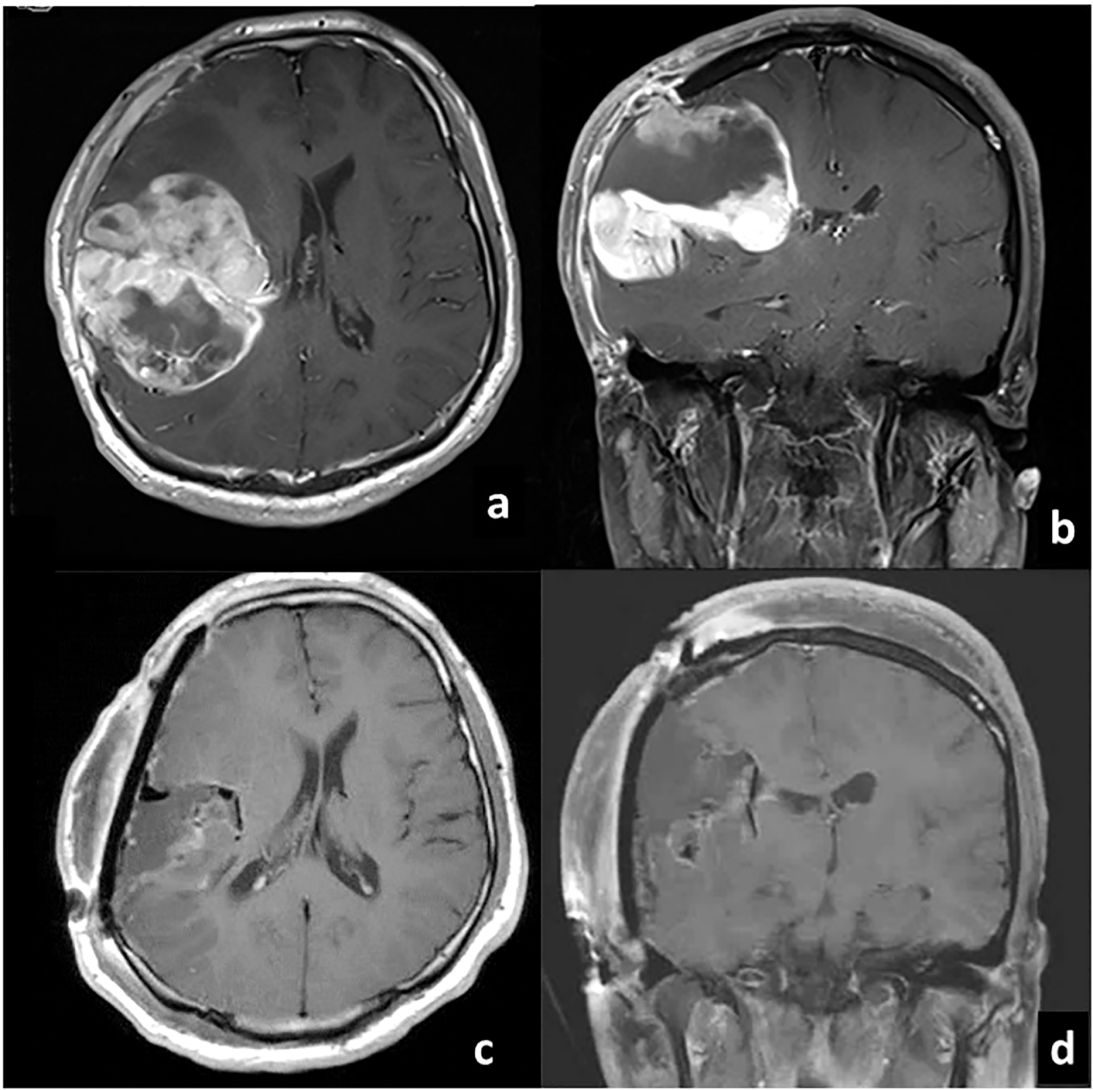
Gadolinium-enhanced T1-weighted MRI before (A) axial, (B) coronal and after (C) axial, (D) coronal) gross total resection of glioblastoma at our institute.
